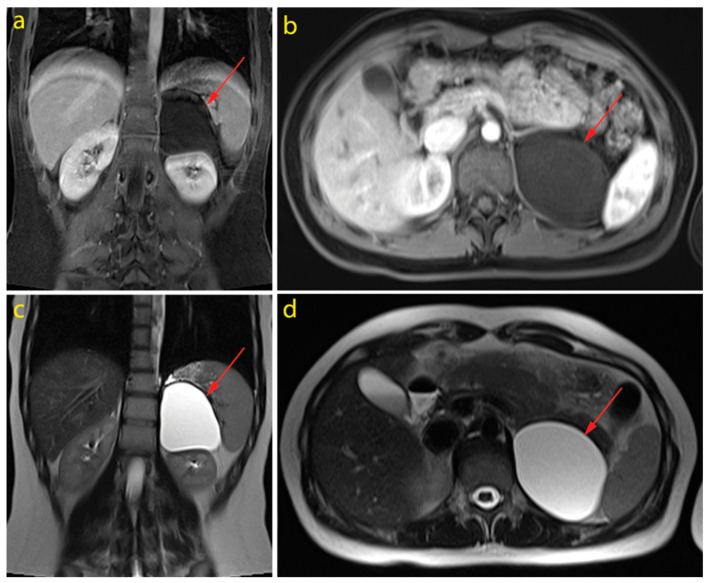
Magnetic resonance imaging (MRI) of the cystic tumour in the left adrenal lodge: T1-weighted sequence (a,b) and T2-weighted sequence (c,d), on the coronary (a,c) and the axial (b,d) plane.
